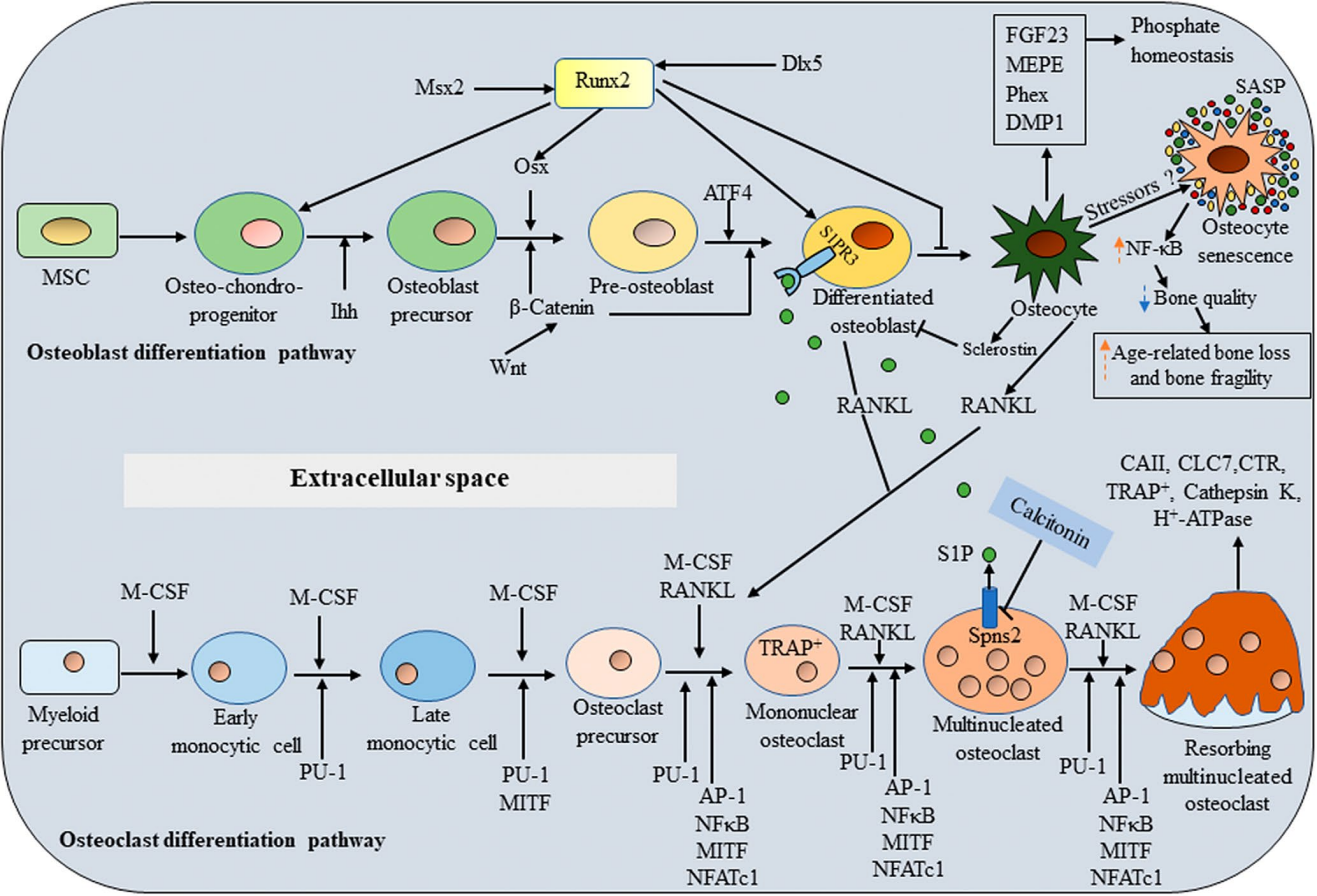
The regulation of bone homeostasis by cellular signalling molecules. Osteoblasts and chondrocytes originate from common MSC lineage precursors. Runx2, Osx, ATF4 are considered master genes for osteoblast differentiation. Ihh and Wnt/β‐catenin are also supporting signalling molecules in osteoblastogenesis. In response to various stressors such as oxidative stress, genomic instability and telomere shortening, osteocytes can be converted into osteocyte senescent cells which have SASP factor. The SASP upregulates NF‐κB and increases pro‐inflammatory factors such as IL‐1α. As a result, senescent cells and the SASP contribute to age‐related frailty and a number of age‐associated chronic diseases including osteoporosis. During osteoclast differentiation, osteoclasts derive from by the fusion of mononuclear progenitors of the monocyte/macrophage family, and osteocytes are non‐proliferative differentiated cells of the osteoblast lineage. M‐CSF and RANKL are essential external stimuli for osteoclastogenesis. PU.1, MITF, NF‐κB, AP‐1 and NFATc1 are essential for differentiation of functional osteoclasts. CT negatively regulates the osteoclast expression of spinster 2 (Spns2), which encodes a transporter for the signalling lipid sphingosine 1‐phosphate (S1P). CT suppresses Spns2 expression and reduces S1P release from osteoclasts. Sphingosine, derived from the membrane lipid sphingomyelin through intermediate ceramide, is phosphorylated to S1P by sphingosine kinases (Sphk1 and 2). S1P is either degraded by sphingosine lyase or secreted through obligatory interaction with Spns2 which is reduced by calcitonin. S1P acts through receptor S1PR3 in the osteoblast to increase osteoblast differentiation and bone formation. The osteocyte (stellate shaped blue cells on the right) expresses several membrane receptors including receptors including PTH and others and controls both osteoblast and osteoclast functions though sclerostin and RANKL. Osteocytes also secrete factors involved in phosphate homeostasis.
